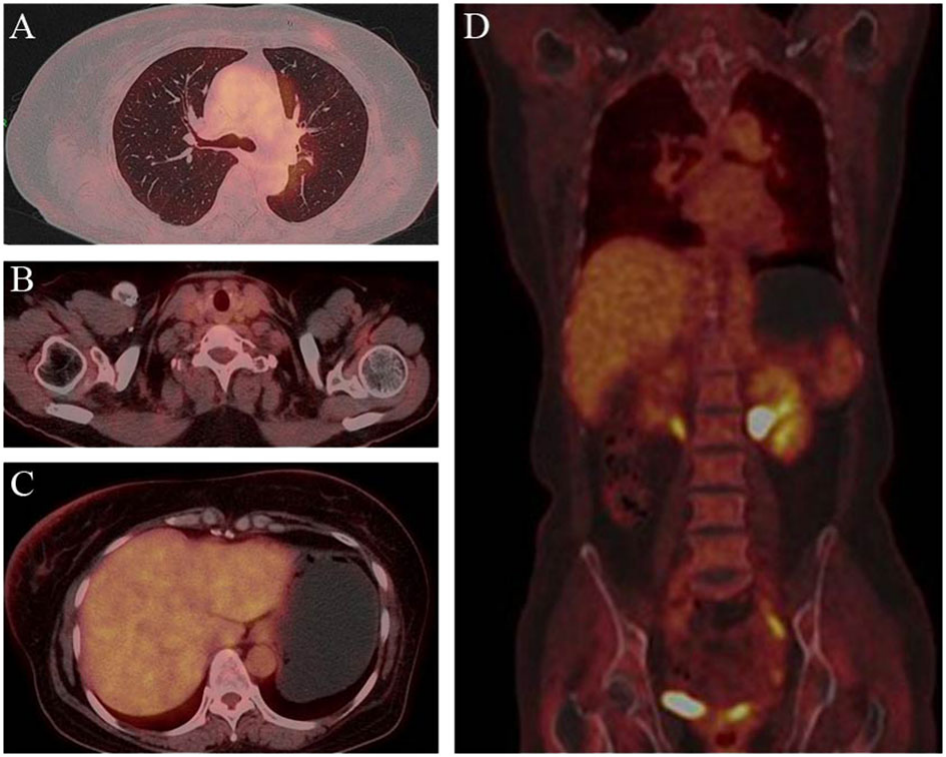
Post-treatment PET/CT findings. PET demonstrated clearcut accumulation of 18F fluorodeoxyglucose in lung (A), lymph nodes in the clavicular region (B), liver (C) and whole body (D).
